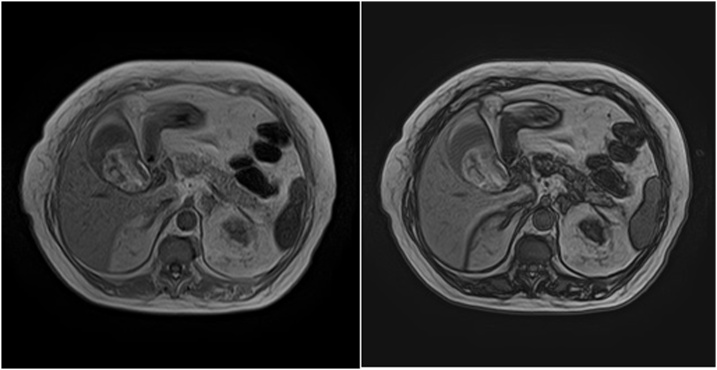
T1 MRI images showing a heterogenic in-phase and out-of-phase hyperintense content of the gallbladder and a thin and homogeneous gallbladder wall.
